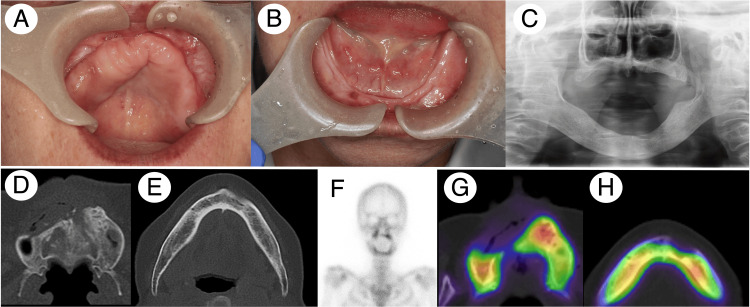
Patient 2 clinical and radiographic findings after the treatment. Intraoral examination revealed no signs of bone exposure or cavity remaining in the alveolar area (A and B). Panoramic X-ray imaging identified the smooth edge of the affected bone without any abnormal osteolytic or sclerotic findings (C, D, and E). Bone scintigraphy and SPECT showed a mild reduction of 99mTc-MDP/HMDP accumulation in the affected areas (F, G, and H). SPECT, single-photon emission computed tomography; 99mTc, technetium-99m; MDP, methylene diphosphonate; HMDP, hydroxy-methylene diphosphonate
